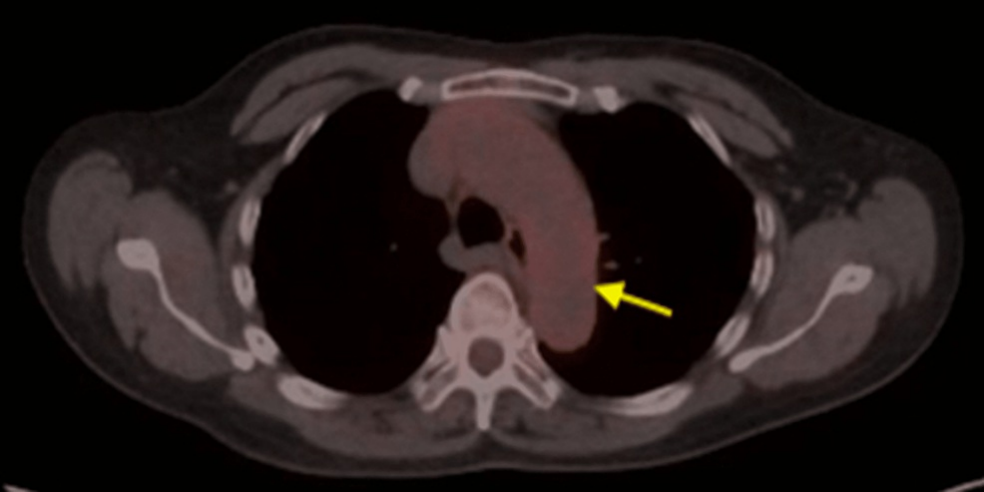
FDG-PET/CT of the chest Yellow arrow: Mild FDG uptake along the thoracic aorta FDG: fluorodeoxyglucose; PET: positron emission tomography; CT: computed tomography
